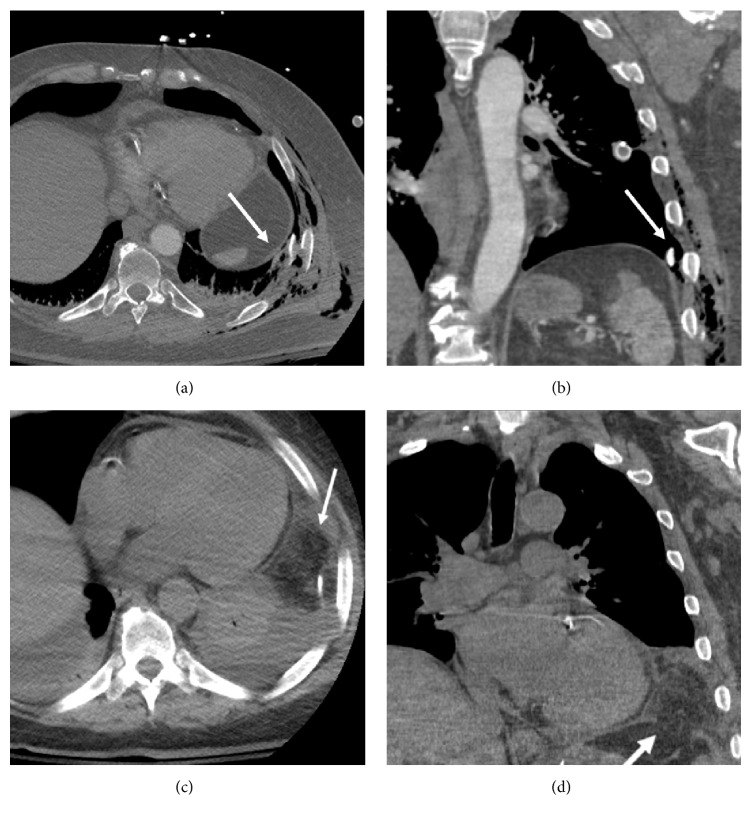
Fractured rib sign in a 59-year-old man after blunt trauma. (a, b) Contrast-enhanced chest MDCT (axial and coronal planes) shows left 10th-rib fracture abutting the diaphragm (fractured rib sign). The diaphragm appears intact on initial trauma CT. (c, d) Repeat chest MDCT (axial and coronal planes) 5 days later reveals new herniation of omental fat (arrows) into the left hemithorax due to subacute diaphragm rupture. Note discontinuity of the diaphragm (d).
